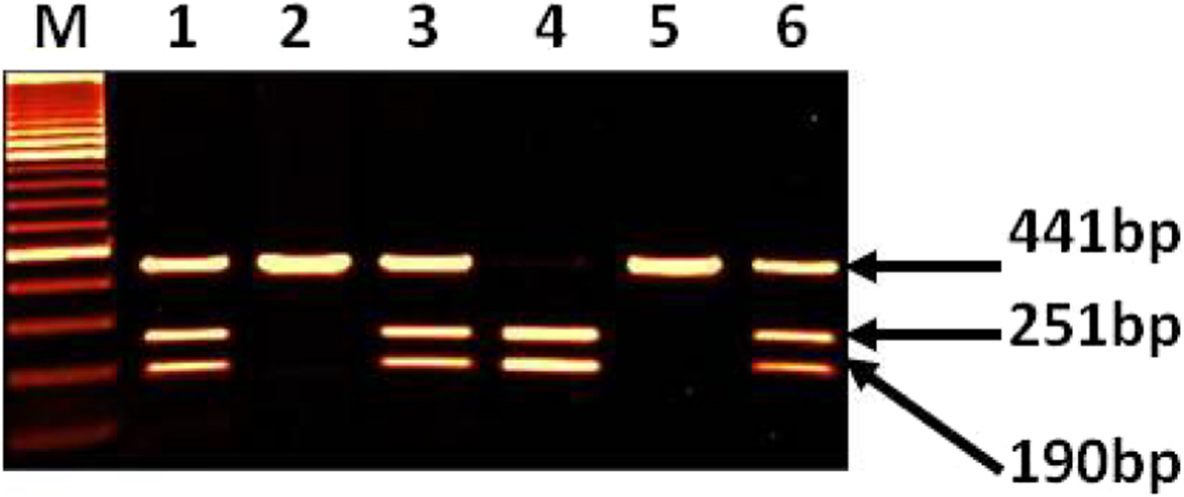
Amplified DNA digested with Bsu36 I showing genotypes of TGF-β1 (509C/T). Lane M: 100 bp DNA marker, Lane 1, 3 and 6 for genotype CT(3 bands of 441, 251and190bp), Lane 2 and 5 for genotype TT (uncut DNA of 441 bp), Lane 4 for genotype CC (2 bands of 251 and 190 bp)
